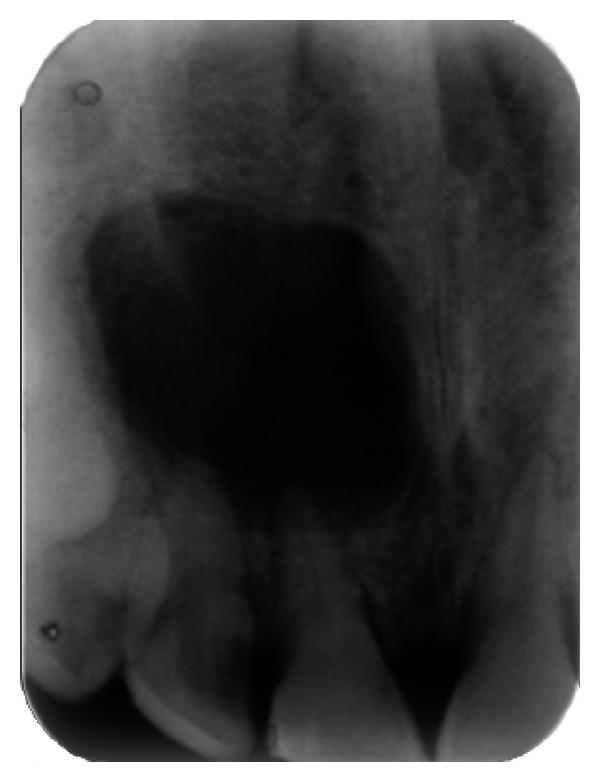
Pre-operative IOPA radiograph showing well-defined large periapical lesion.
